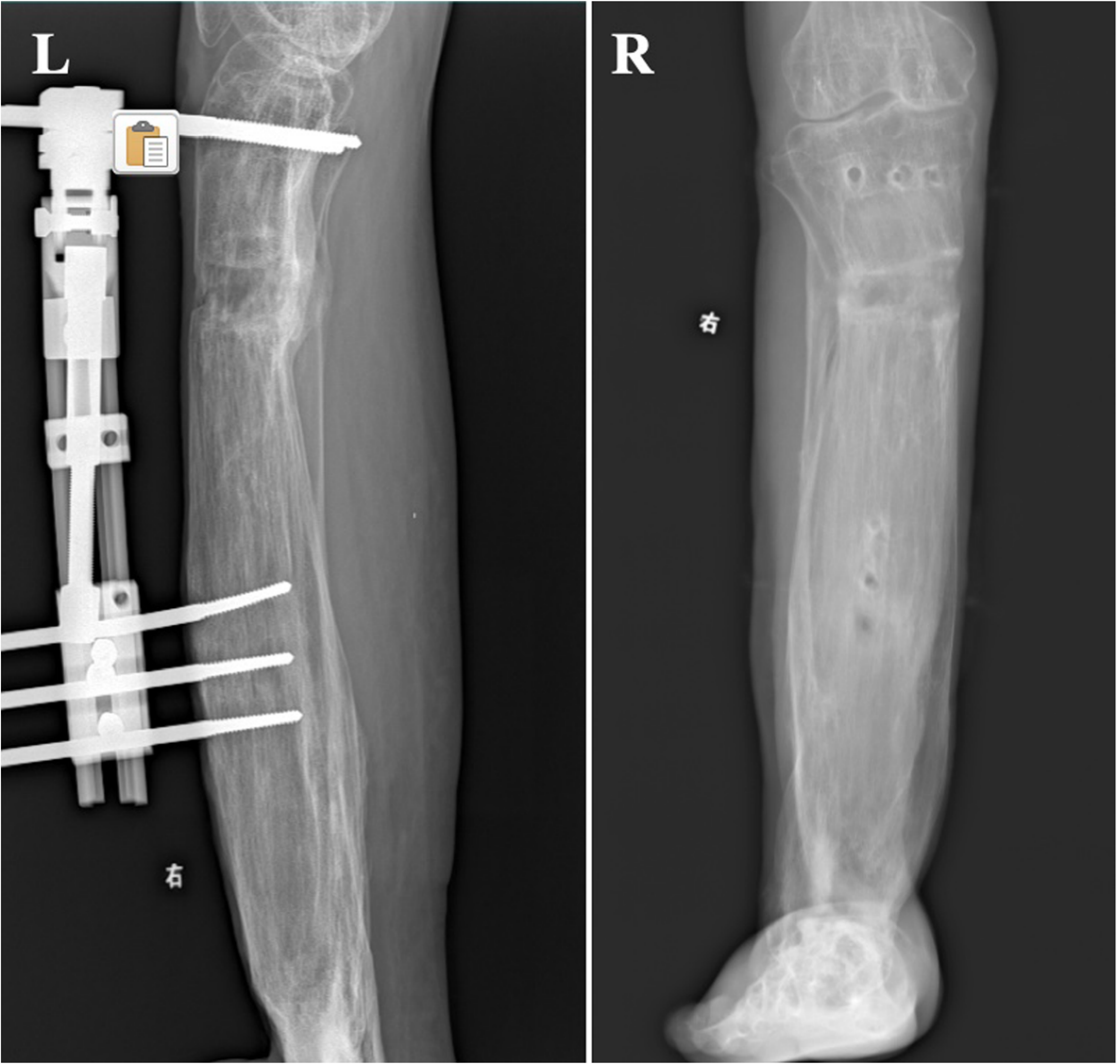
L, lateral view X-ray of right lower leg after completion of valgus correction and distraction. R, AP view X-ray of right lower leg after removal of apparatus
